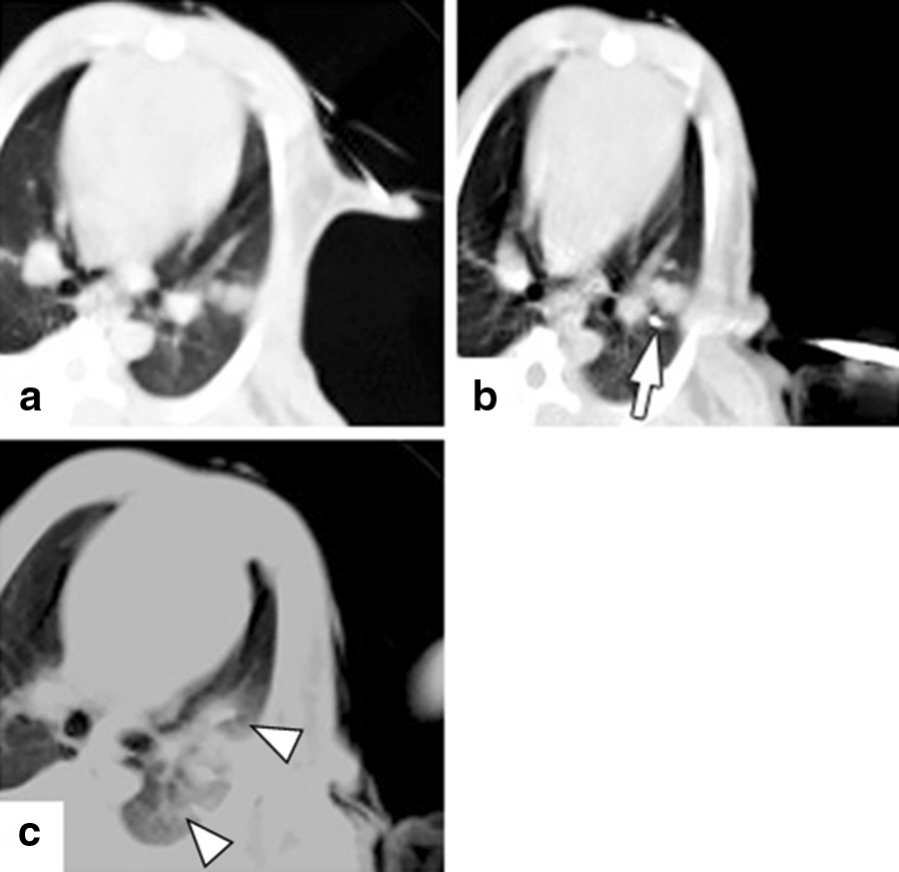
a CT image of VX2 tumor before RFA. A round VX2 tumor with a well-defined boundary is seen in the left lower lobe. b CT image of VX2 tumor during RFA. A LeVeen Needle is puncturing the tumor, and the electrode’s tip (arrow) has been expanded. c CT image of VX2 tumor immediately after RFA. A ground glass opacity (arrowhead) is observed around the tumor, confirming its ablation. The electrode’s tips are seen
